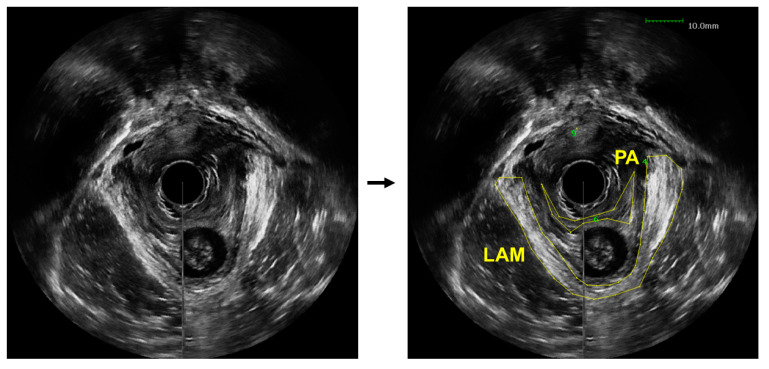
An axial view image obtained from a 3D endovaginal ultrasound image acquired from a patient with pelvic floor prolapse (left). Levator ani muscle (LAM) and puboanalis (PA) were traced by an urogynecologist using the BK viewer software (right).
